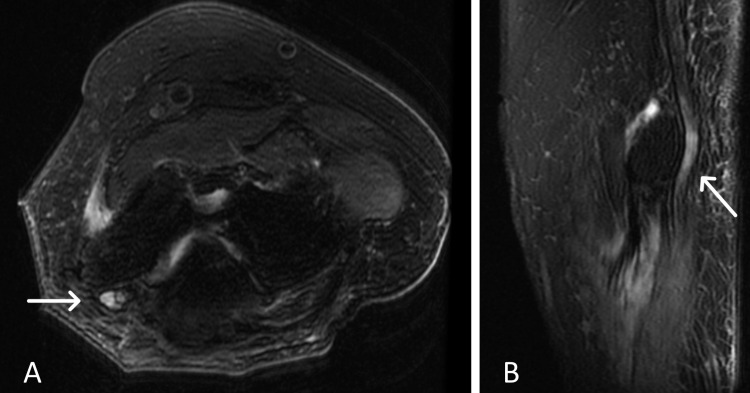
Axial (A) and Sagittal (B) MRI of the left elbow reveals thickening and increased signal intensity of the ulnar nerve proximally and at the level of the cubital tunnel, consistent with ulnar neuritis/cubital tunnel syndrome.
